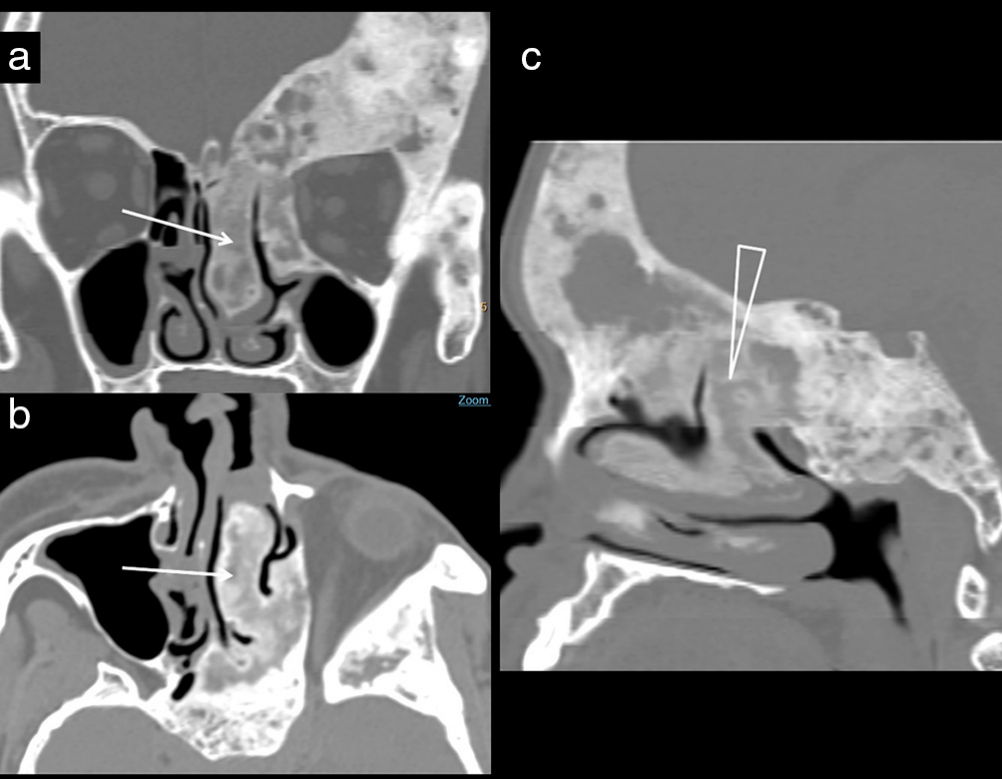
A 32-year-old adult female with occasional nasal bleeding. (a) Coronal CT image at the mid-nasal cavity level demonstrates thickening of the orbital plate and zygomatic bone. There is massive thickening of the lateral lamella and middle turbinate (arrow), encroaching upon the superior aspect of the left nasal cavity. (b) Axial image demonstrates an enlarged superior turbinate showing posterior continuity with the skull base (arrow), demonstrated better on the sagittal CT reconstruction (triangle; c).
